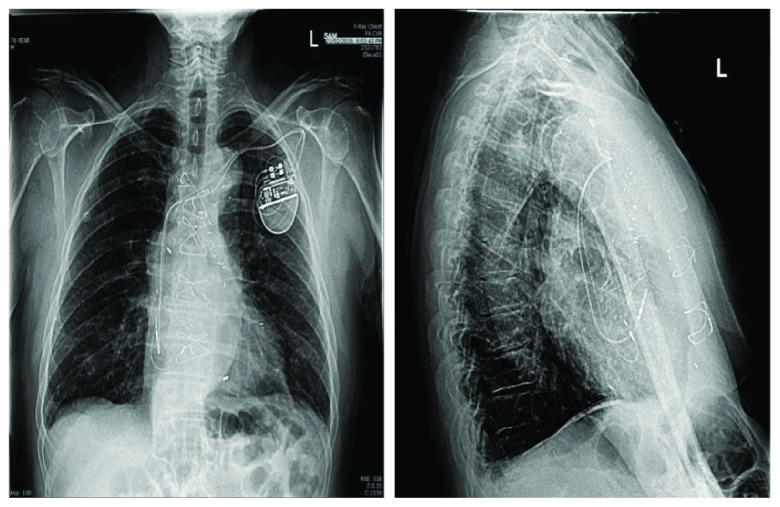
Chest X-rays (posterior-anterior and lateral views) after transcatheter aortic valve implantation and His-bundle pacemaker insertion.
